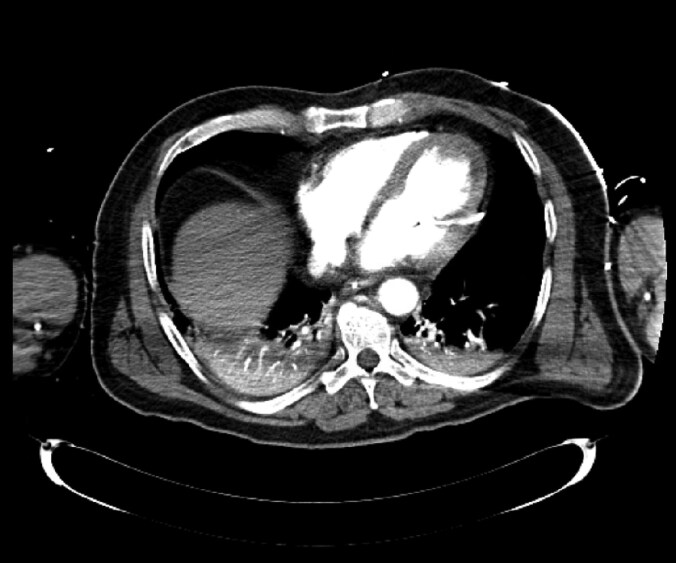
Axial CT scan demonstrating left ventricular (LV) perforation secondary to Impella® CP placement.
